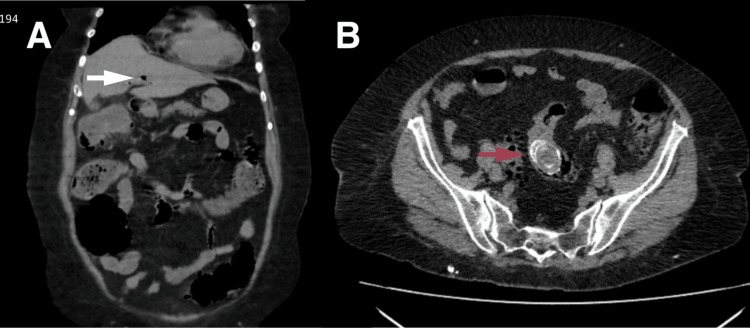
Abdominopelvic computed tomography. (A) Axial section showing gas within the intrahepatic biliary tree (pneumobilia) (white arrow). (B) Axial section identifying a hyperdense intraluminal image corresponding to an ectopic gallstone impacted in the sigmoid colon (red arrow). The findings are consistent with Rigler’s triad and are suggestive of gallstone ileus (Bouveret syndrome).
